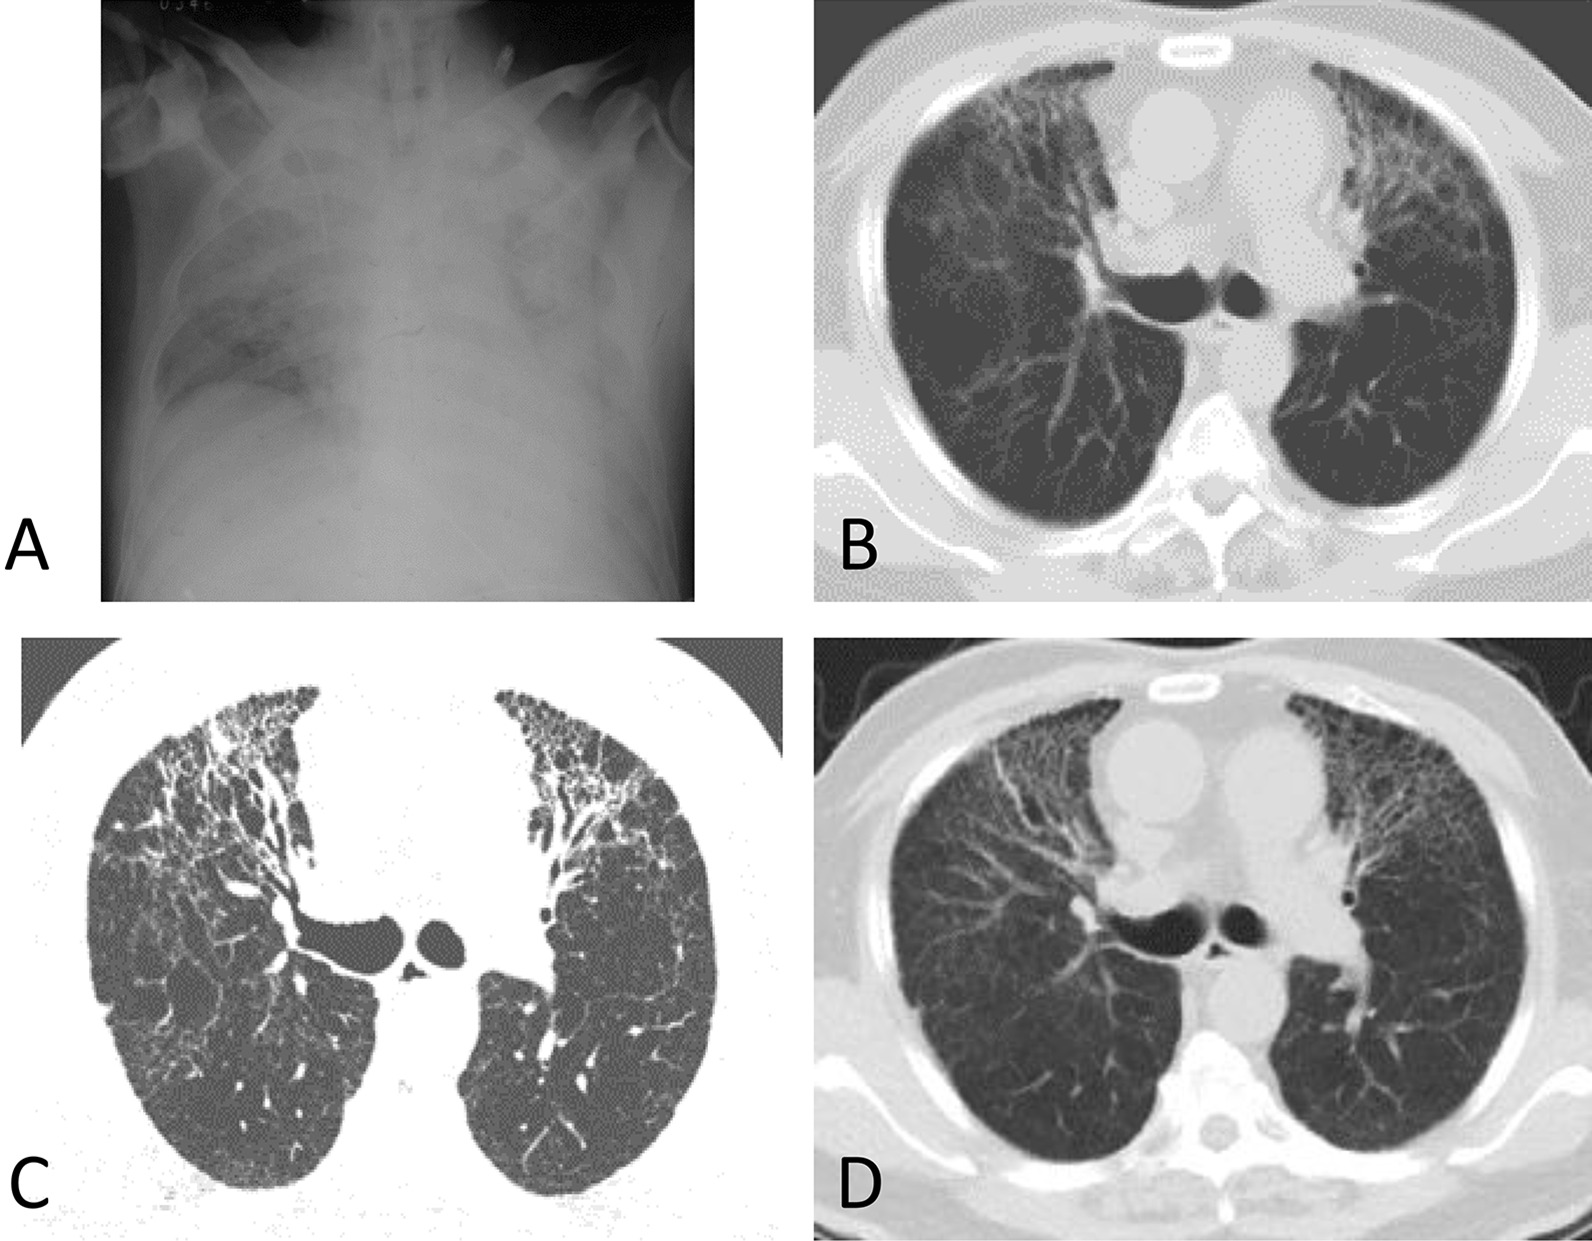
Serial CT images of a male SARS survivor in 15 years. A Showed diffused consolidation in bilateral lungs on June 14, 2003. B–D Showed a stable reticular interstitial fibrosis with tractive bronchiectasis in the serial follow-up, after 1 year (B, November 2004), 3 years (C, August 2006) and 15 years (D, March 2018) of SARS pneumonia
